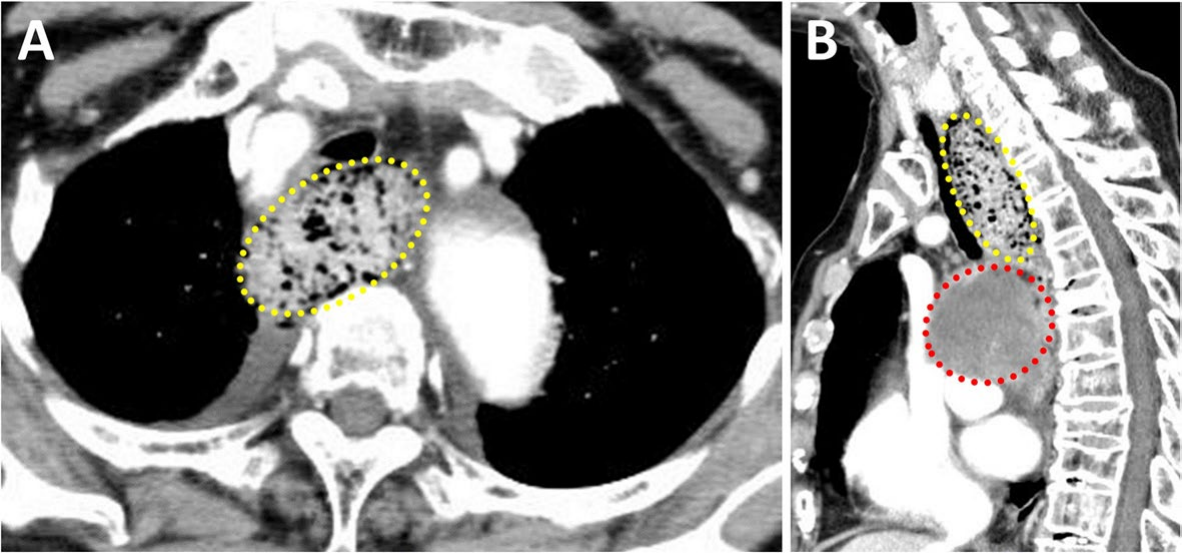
Axial section (A) and sagittal section (B) of preoperative thoracic computed tomography (CT). There is a significant amount of food residues in the upper thoracic esophagus (yellow dotted line circle) (A, B). Thoracic aortic aneurysm with impending rupture (red dotted line circle) is compressing the thoracic esophagus with food residues
